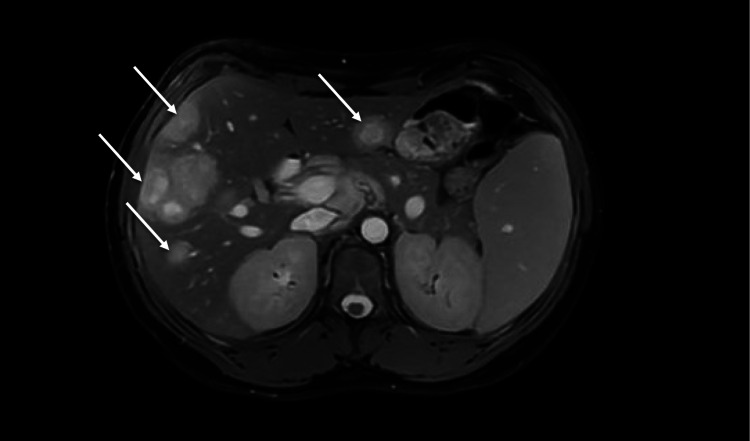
MRI of the liver with and without contrast demonstrating several variable sized lesions in the liver with a nonspecific appearance, representing either multiple abscesses or necrotic metastases (demarcated by arrows).
